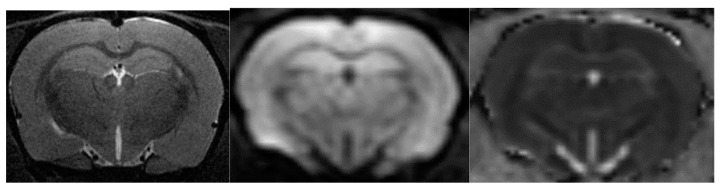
From left to right are images of T2-WI, DWI, ADC.
